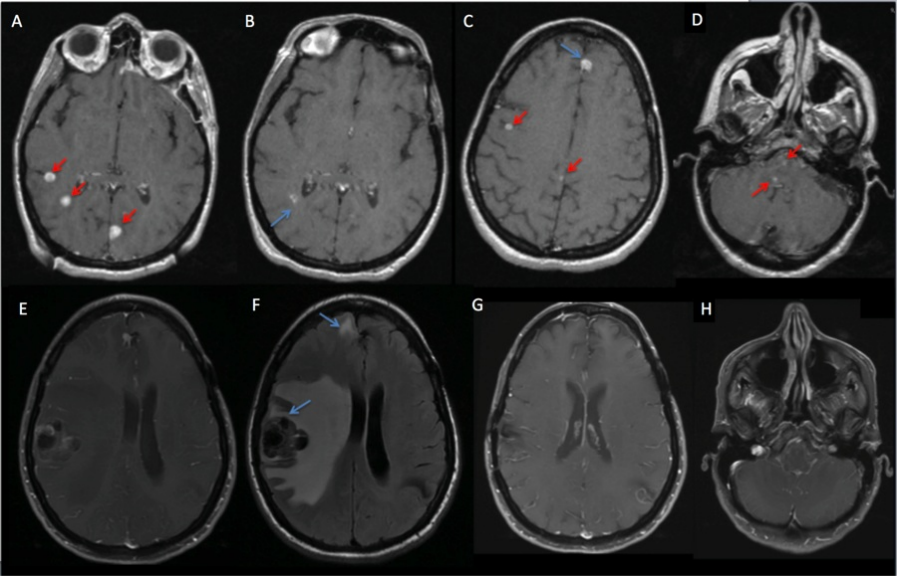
Patient MK - MRI of the brain. Patient MK initially presented in 2013 with one resection cavity and A) three untreated lesions. On her September 2014 GK MRI, her treated lesions were stable (B, blue arrow)—including a lesion treated in January 2014 (C, blue arrow); however, she presented with nine lesions, four of which are shown here (C, D, red arrows), which were treated with 20 Gy to various isodose lines, though two brainstem lesions were treated with a lower dose of 18 Gy to minimize the risk of brainstem injury (D, red arrows). In March 2015, she had persistent enhancement of a lesion (E) with surrounding edema (F), which underwent surgical resection and demonstrated persistent disease. On the most recent scan, all lesions are resolved or stable (G, H). MRI - magnetic resonance imaging, GK - Gamma Knife.
